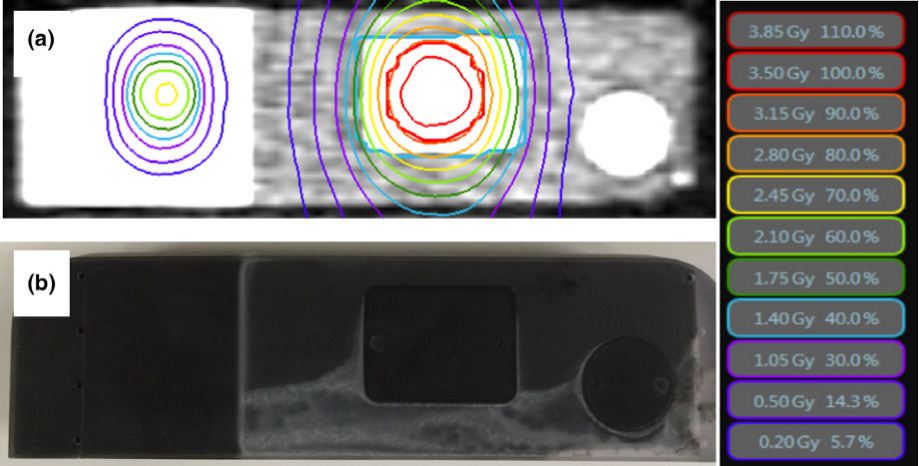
Target rod cross‐section MRI image with isodose distribution superimposed (a) and optical digital camera image (b). The reference beam appears on the left and the PTV on the right in this view. The PTV is a 3 cm diameter spherical target centered about the cuboid gating target.
